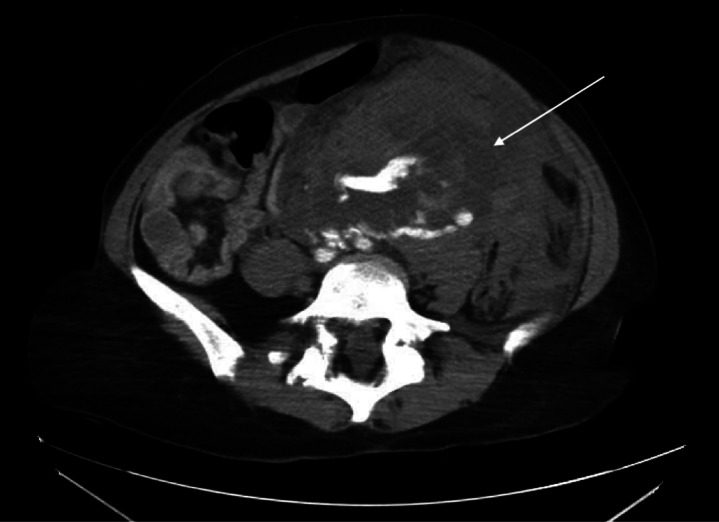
Cross-sectional image of abdominal computed tomography showing hematoma with extravasation of contrast (arrow) as a result of ruptured left renal artery aneurysm.
